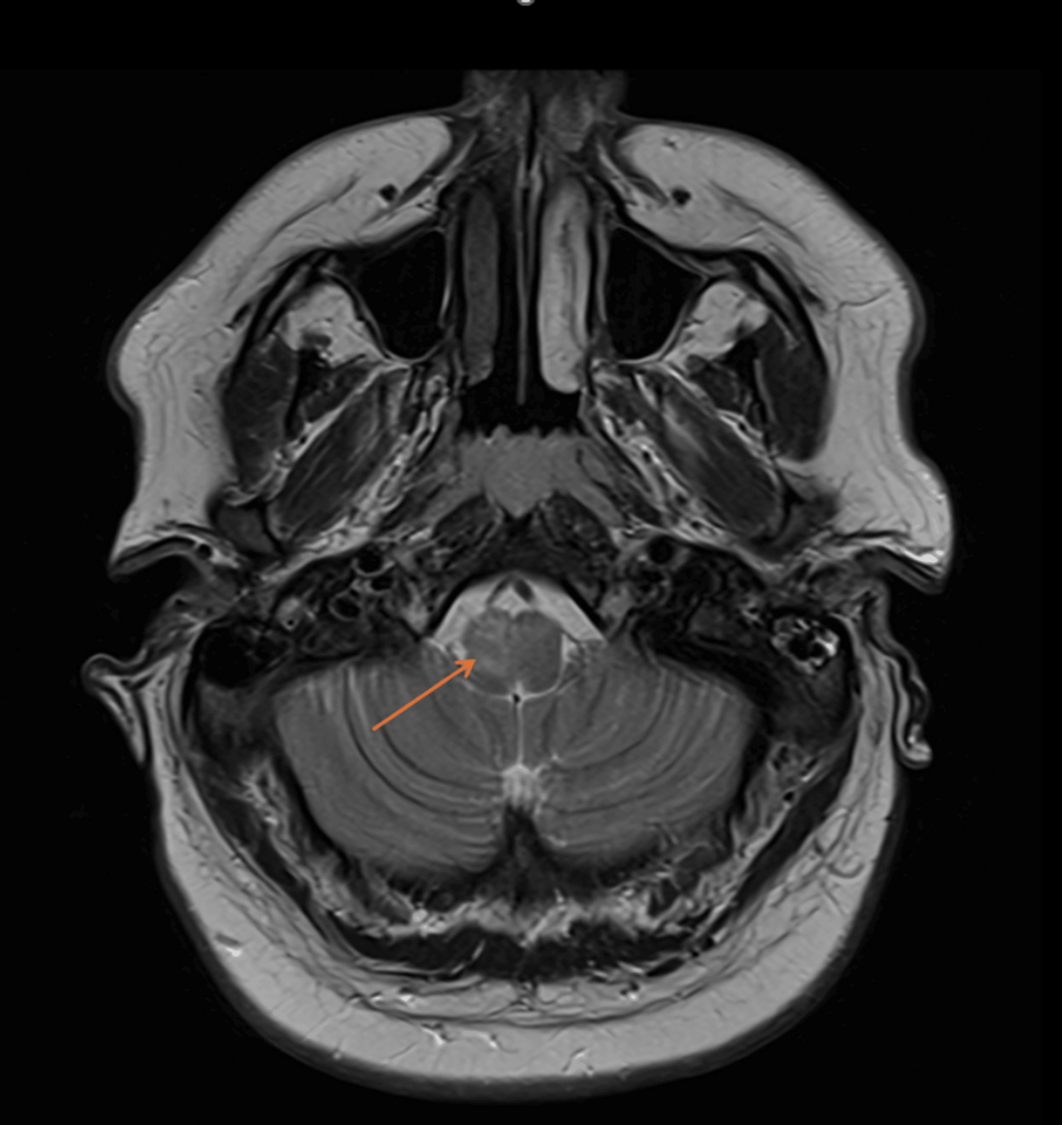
Axial T2-weighted MRI brain with contrast demonstrating a subtle area of high signal in the right medulla (arrow), without associated enhancement, consistent with inflammatory involvement in rhombencephalitis and correlating with the patient’s clinical presentation.
